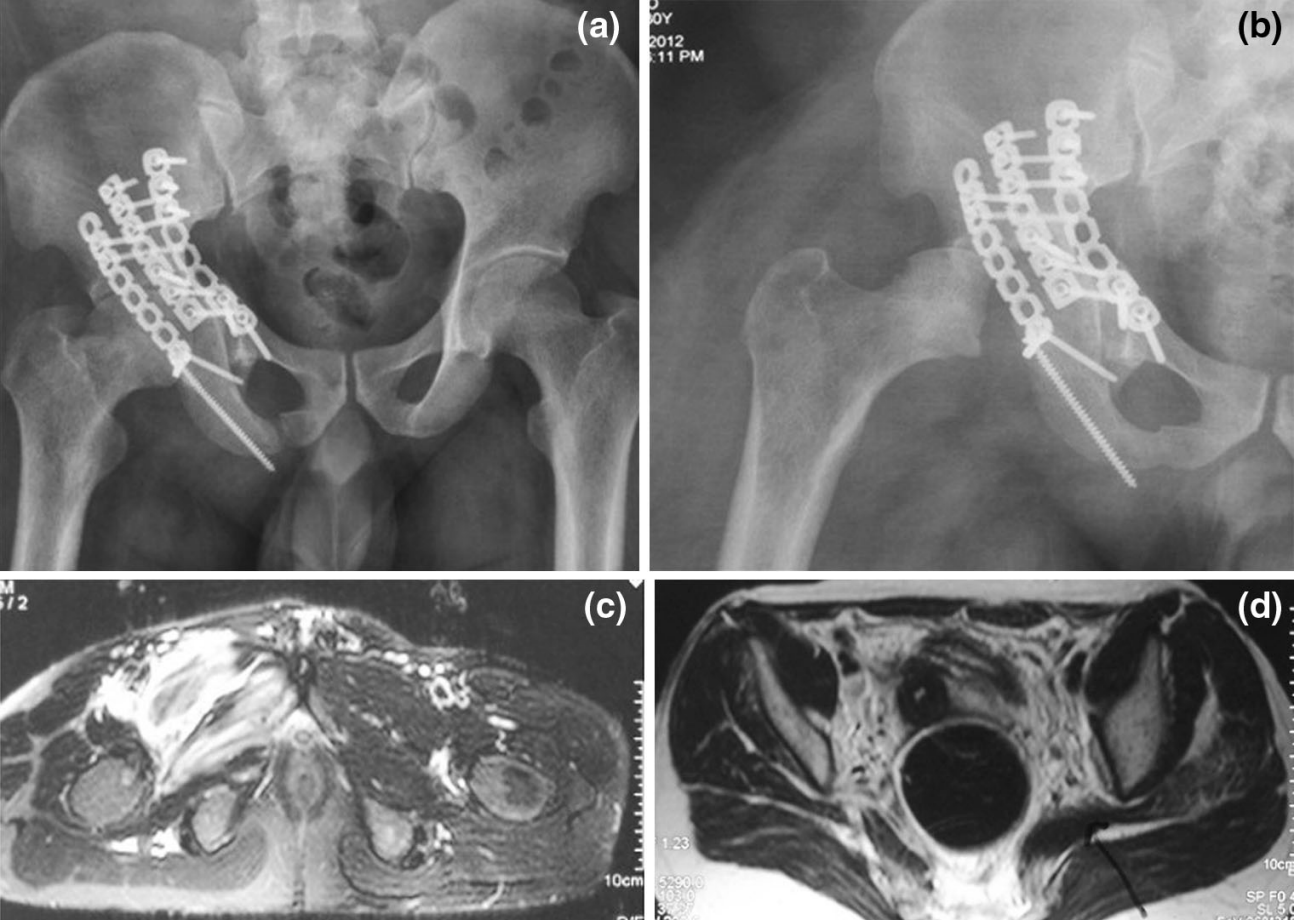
Imaging of case 3 showing T-type acetabular fracture. a 2-month post-operative antero-posterior radiograph showing operative fixation of the fracture with reconstruction plates and normal femoral head; b 4-month post-operative radiograph showing features suggestive of avascular necrosis of femoral head with resorption; c pre-operative MRI of the patient had shown a complete tear of obturator externus; and d a partial tear of piriformis
